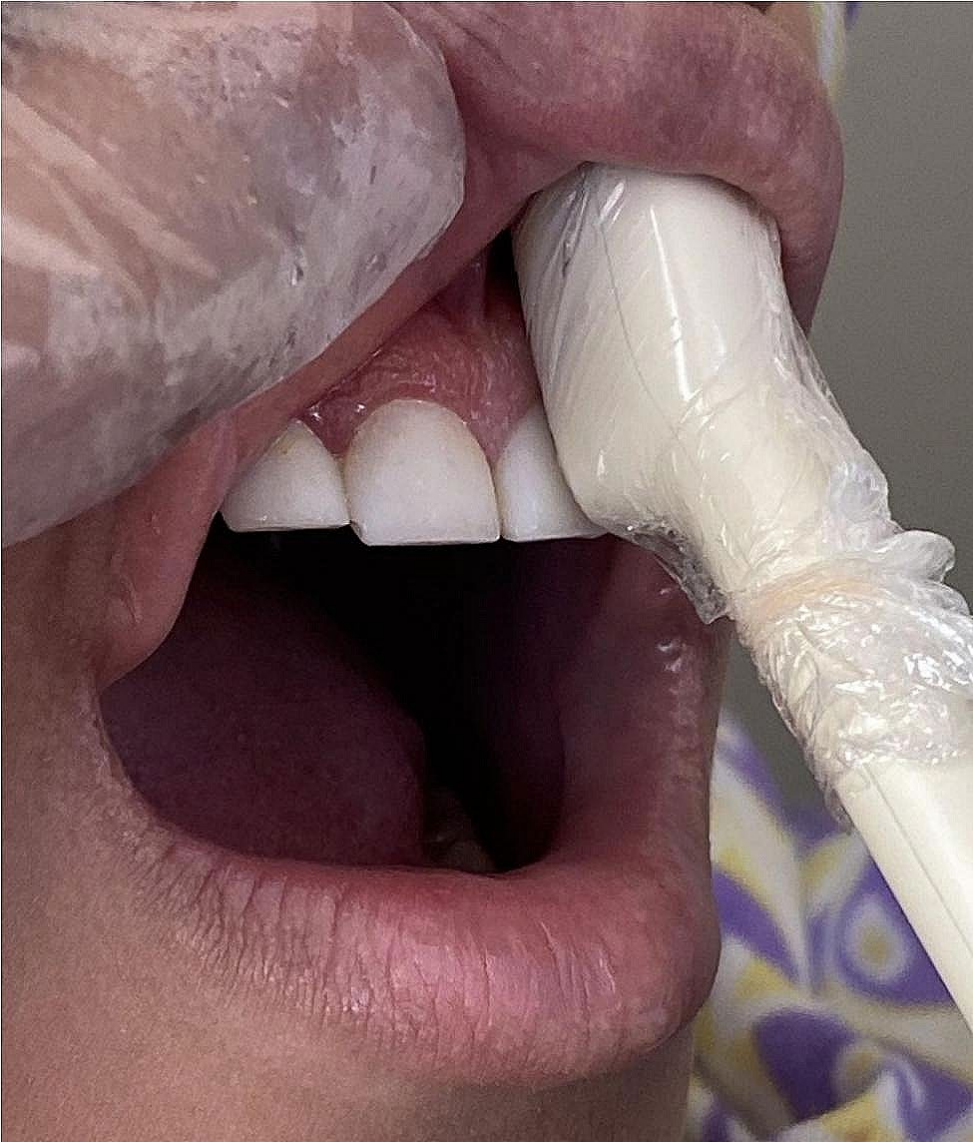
Positioning of intraoral probe of ultrasound for measurement of gingival thickness
